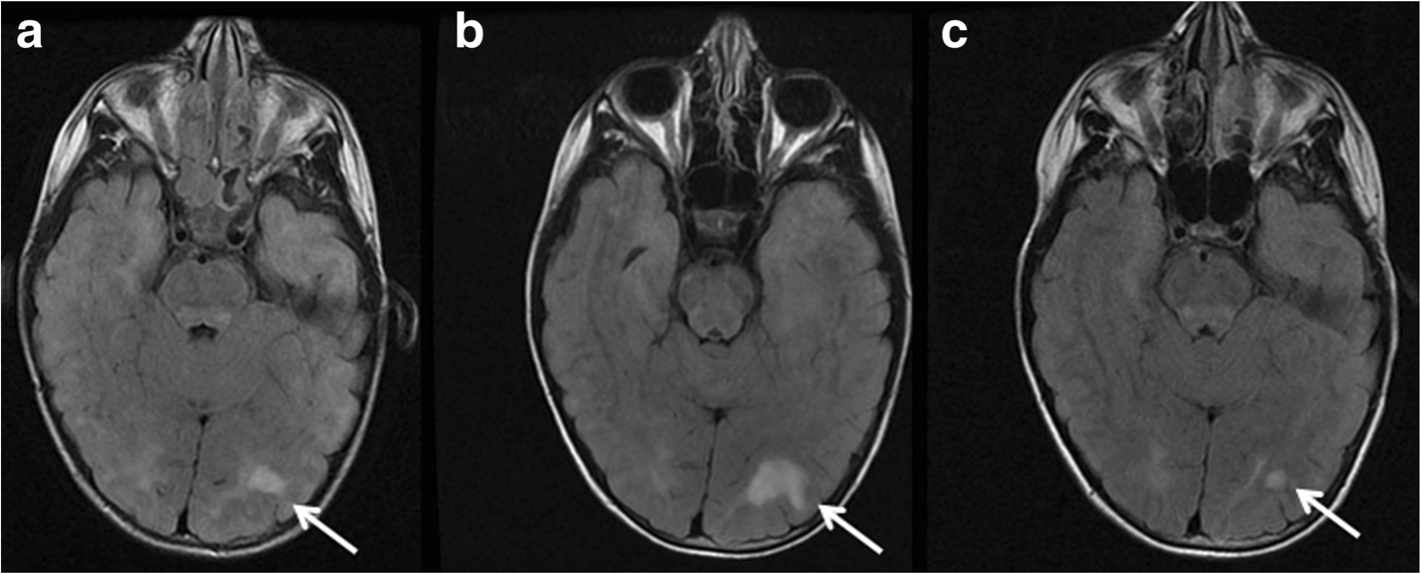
The figure shows pattern B (see text) on axial brain MRI in a patient with NF1. Bilateral occipital abnormal signal intensities are evident. The left occipital abnormal signal intensity (arrow) increased in size from age 4 years (a) to age 7 years (b). It then decreased in size and stabilized at age 9 years (c)
